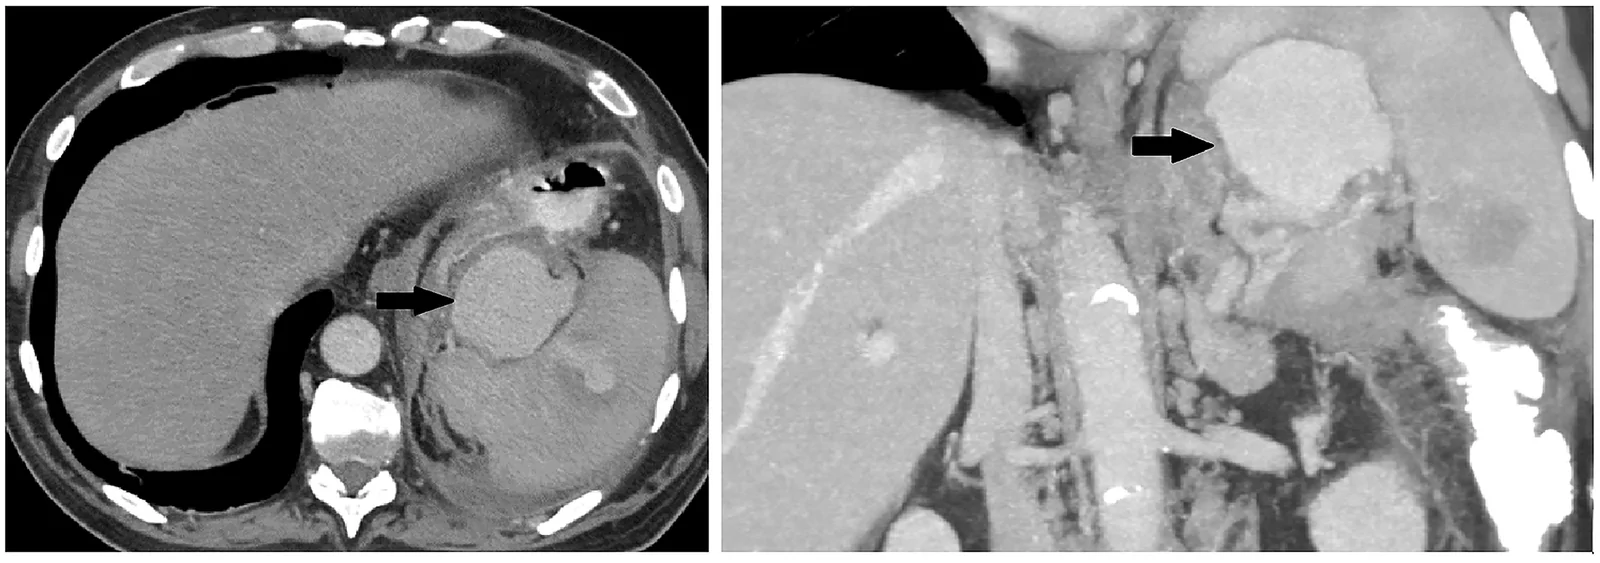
Portal venous-phase contrast-enhanced CT performed on August 21, 2023, showing marked enlargement of the splenic hilar lesion to 54 mm (arrows), with prominent enhancement during the portal venous phase. The persistence of contrast enhancement beyond the arterial phase represented an atypical feature for a classic splenic artery pseudoaneurysm.
